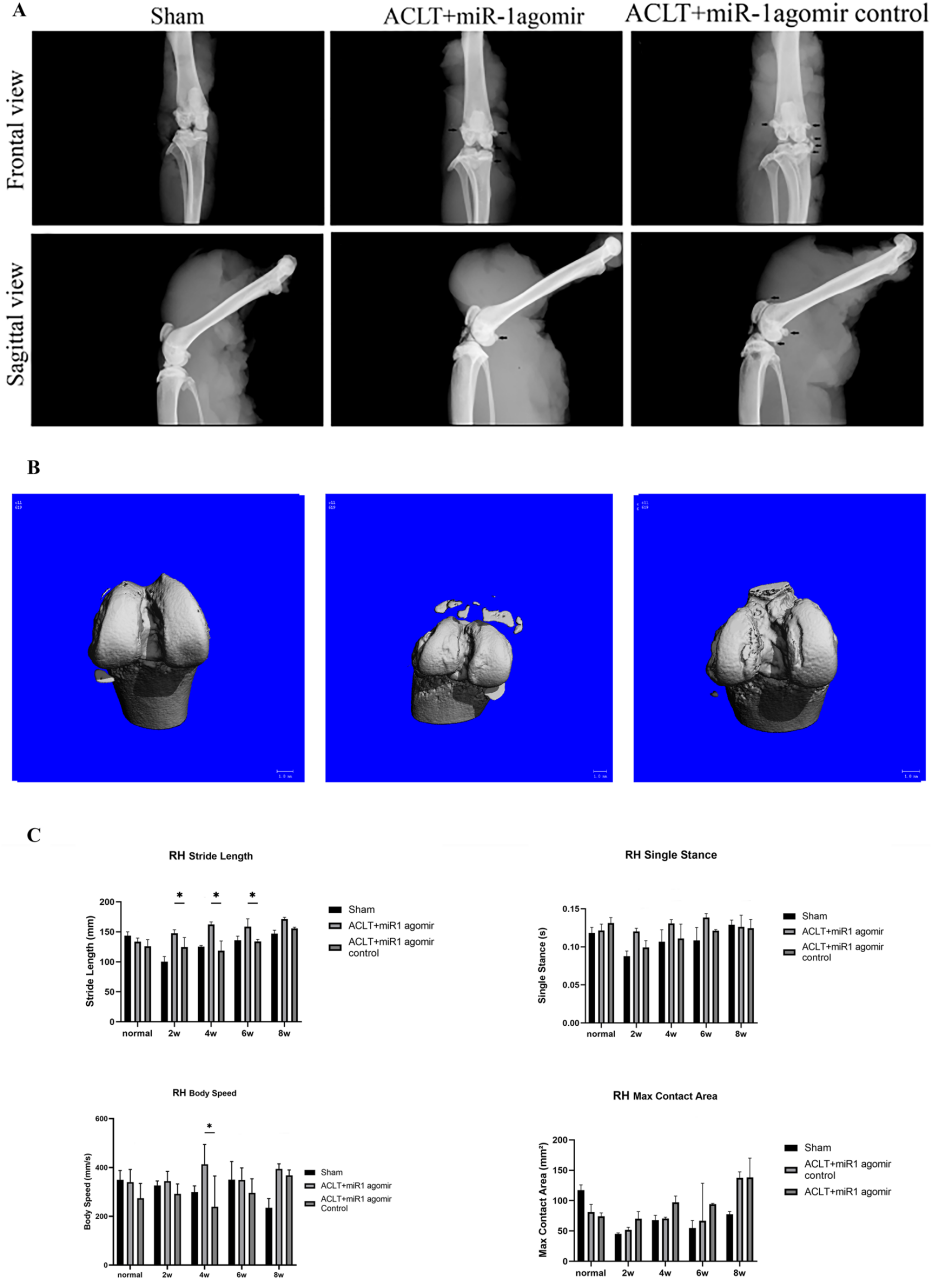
(A) Comparison of osteophyte formation in the frontal and sagittal X-rays eight weeks after ACLT. (B) Comparison of CT detection of osteophyte formation eight weeks after ACLT. (C) Comparison of gait analysis after ACLT. P* < 0.05.
